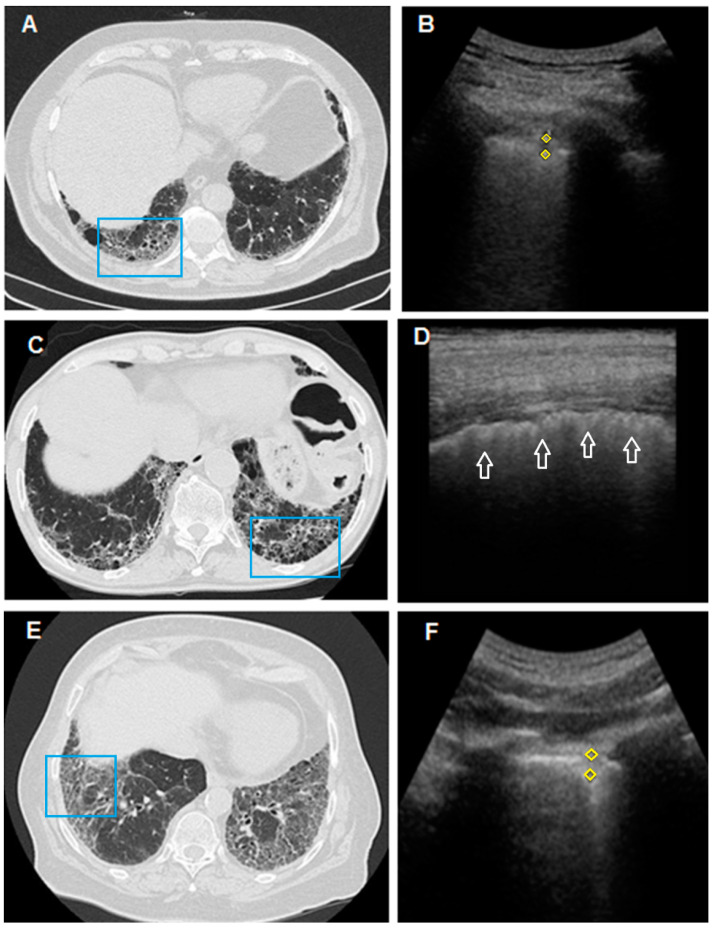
In (A), HRCT scan shows bilateral honeycombing, more severe in the right postero-basal area (blue box); In (B), TUS scan with convex probe (3.5–5 MHz), in the chest area corresponding to the blue box in (A), shows a subpleural hypoechoic area (yellow rhombus) of 4.5 mm (probably a traction cyst). In (C), HRCT scan passing through the postero-basal segments shows honeycombing pattern, larger on the left (blue box). In (D), TUS scan performed with a linear probe (8–12.5 MHz) in the chest area corresponding to the blue box in (C) shows a thickened (>2 mm), irregular, fragmented and blurred hyperechoic pleural line (white arrow), but subpleural nodules are not appreciated. In (E), HRCT scan of the thorax passing through the postero-basal segments shows a typical fibrotic distortion of the pulmonary parenchyma, with ground-glass opacity superimposed on a predominant reticular pattern with subpleural and basal distribution and with associated traction bronchiectasis in the absence of a frank honeycombing (UIP “probable”). (F) TUS examination performed with a convex probe (3.5–5 MHz) probe in the chest area corresponding to the blue box in (E) shows a subpleural hypoechoic nodule of 5.9 mm.
